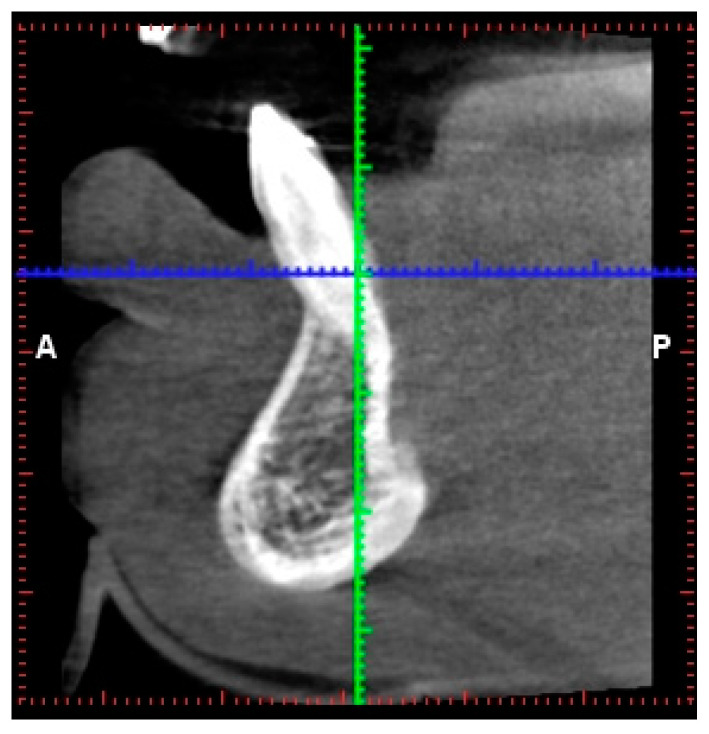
CBCT cross-sectional image of the right lower incisor.
